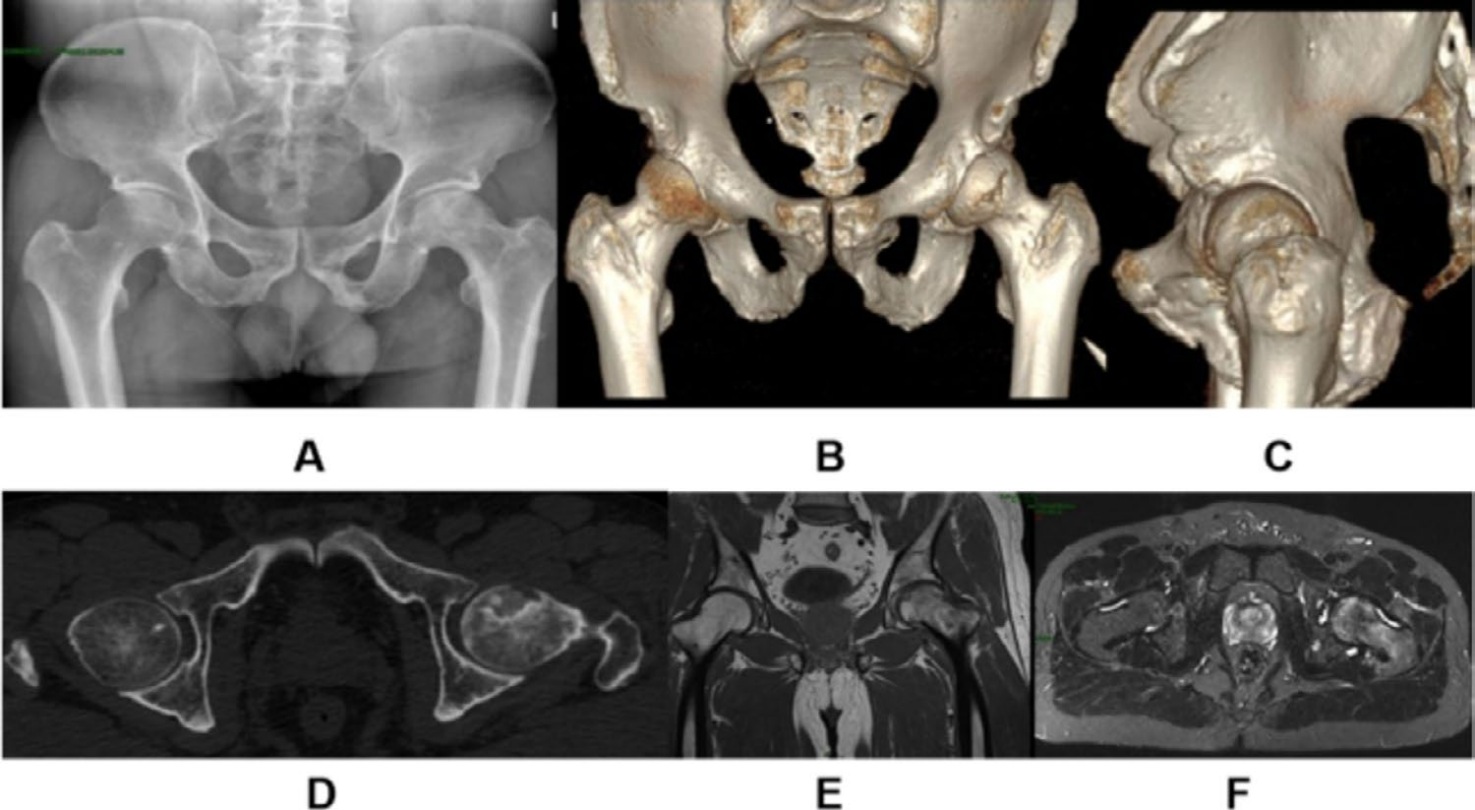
(A~F) Preoperative images of an HIV-positive ONFH patient (bilateral); bilateral hip pain for 3 months, aggravated by limited mobility and claudication for 1 month; bilateral hip ARCO stage IIc, treated with bilateral femoral head drilling and decompression combined with bone grafting and autologous bone marrow transplantation. Wear of the femoral head, narrowing of the joint space and uneven surface of the femoral head (X-ray A); significant necrotic area of the femoral head, wear and deformation, narrowing of the joint space and uneven surface of the femoral head (CT B,C,D); mixed signal within the femoral head, depression of the cartilage surface and narrowing of the joint space (MRI E,F)
